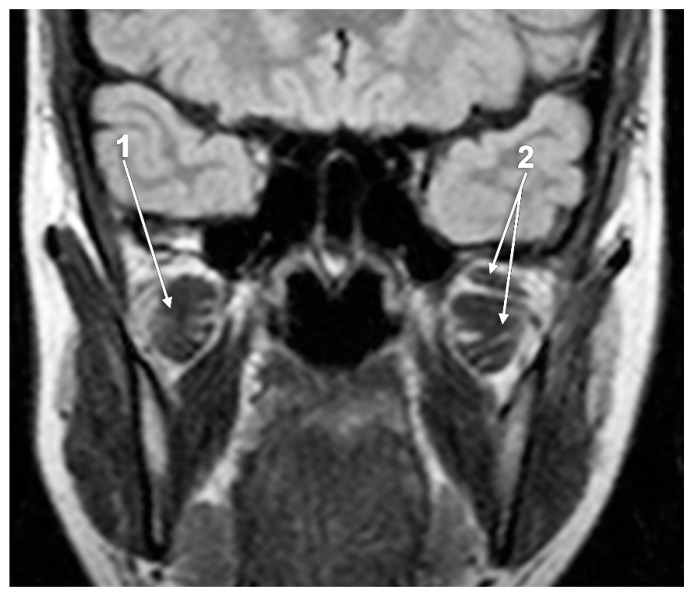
Coronal MRI slice. Bilateral morphological asymmetry of the lateral pterygoid muscles, single-headed (1) and double-headed (2).
